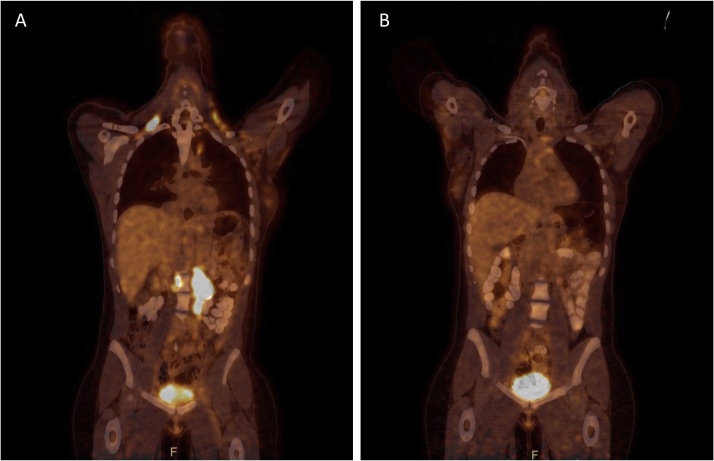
PET CT for assessment of extension showing multiple gangliotic supra and infra diaphragmatic hypercaptation (A) corresponding to metastatic adenopathy. Control PET CT in November 2018 showing the absence of tumoral recidive (B).
